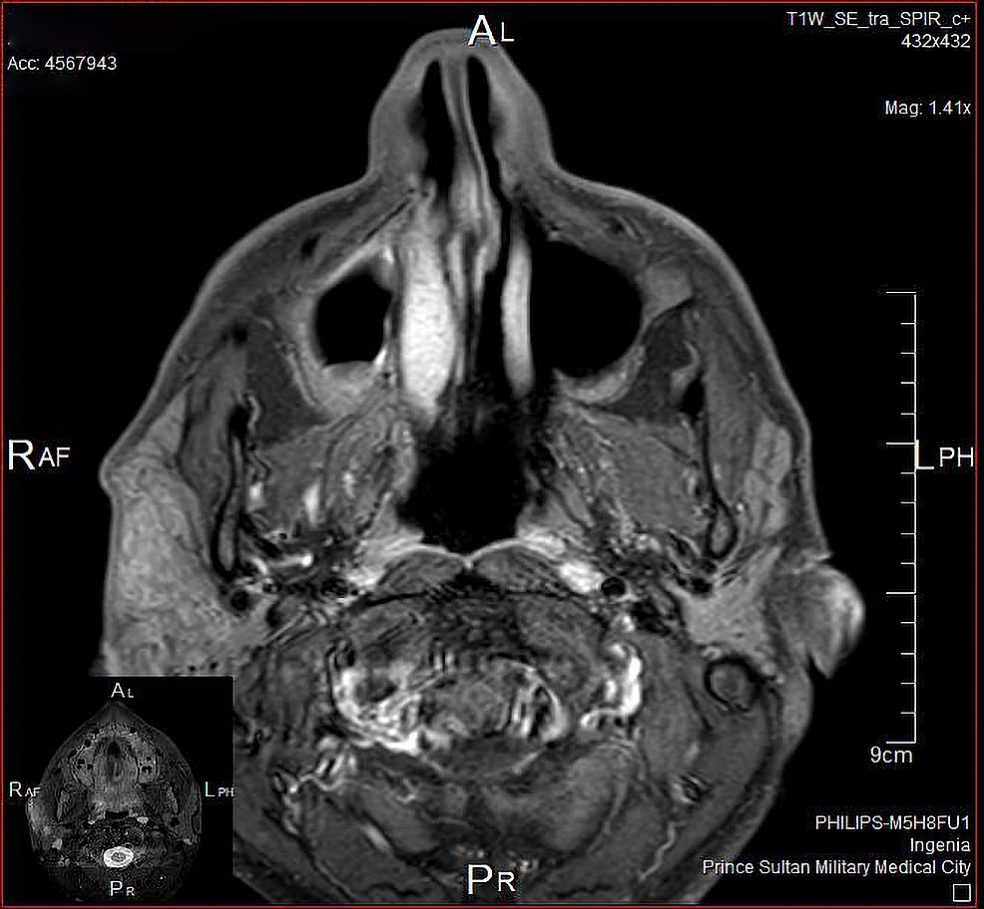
MRI showing infiltration of the overlying subcutaneous tissue - image 1 MRI: magnetic resonance imaging
